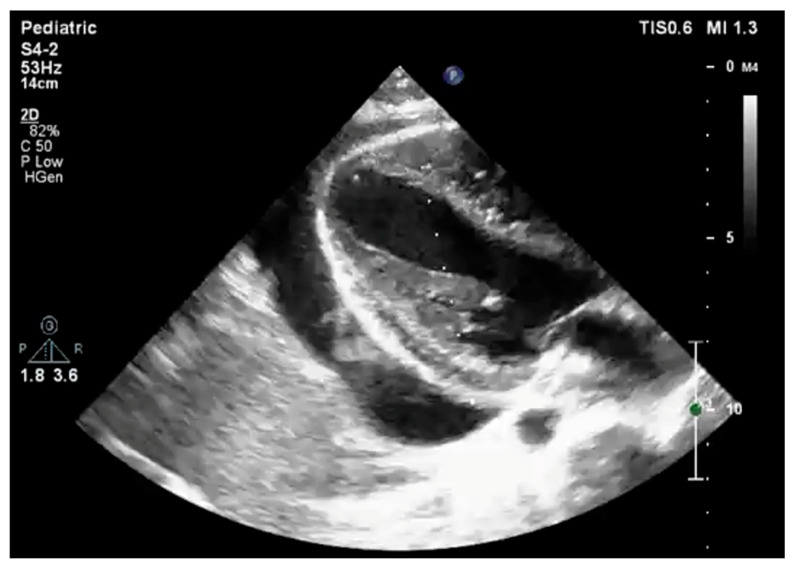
Echocardiographic parasternal long-axis view during the fourth relapse of pericarditis showing severe pericardial effusion.
